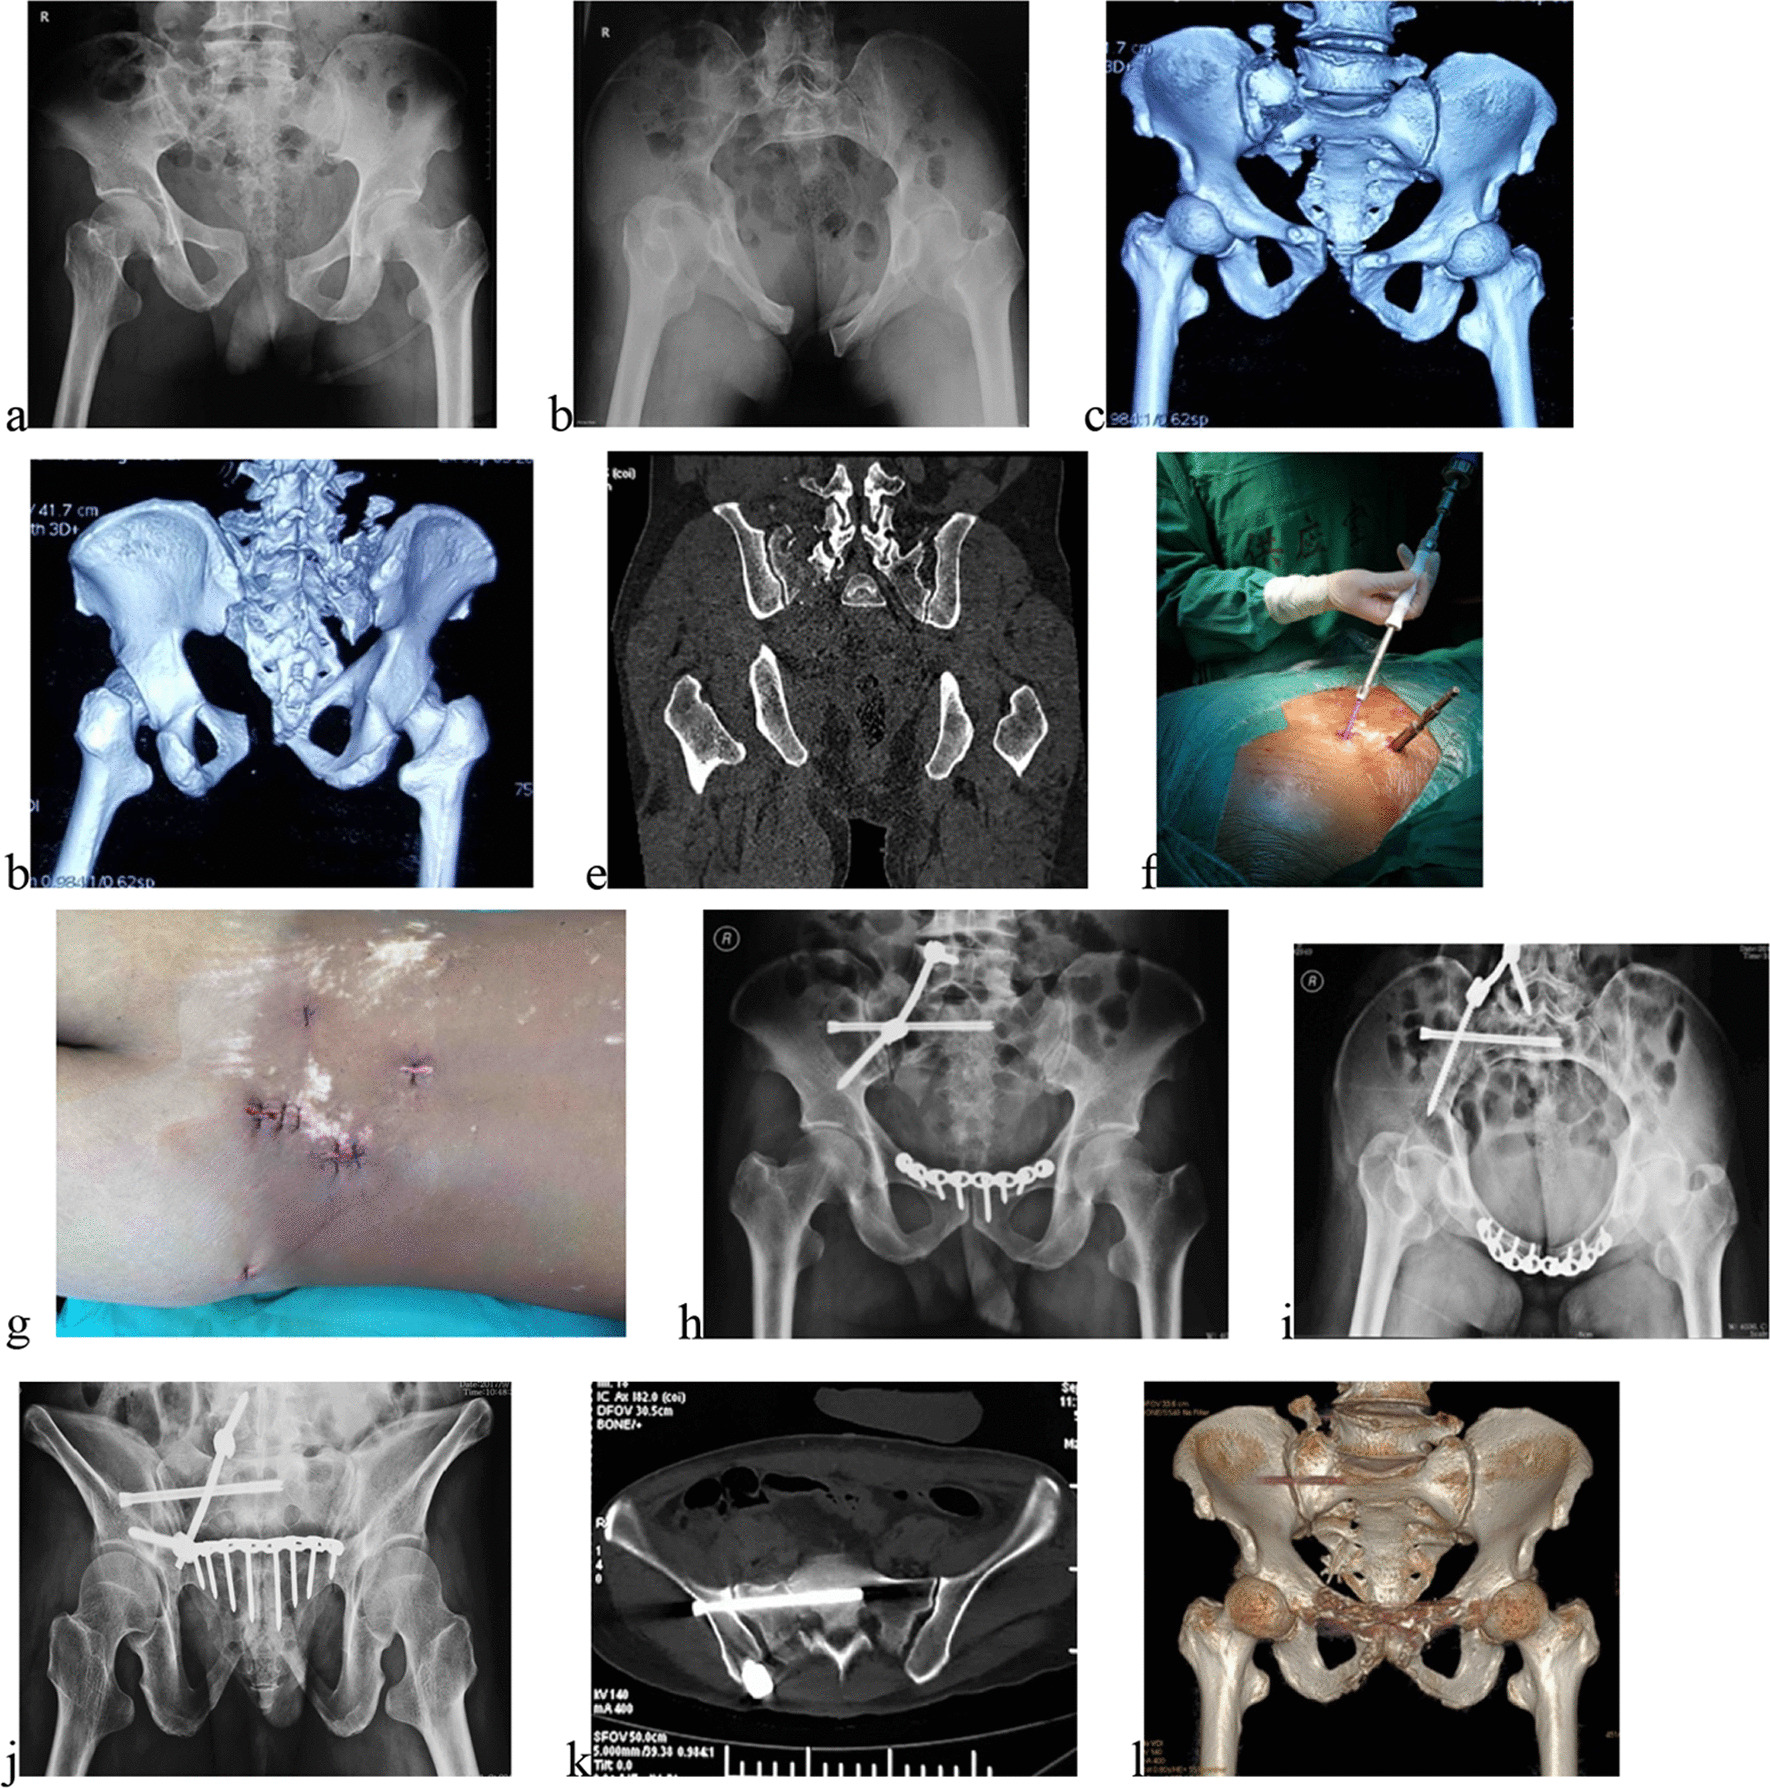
Male, 48 years old, a fall injury. a, b Anteroposterior and inlet pelvic views after admission. c, d 3D CT reconstruction: a Tile C1 pelvic ring injury. e The coronal view showing the right S1 facet fracture. f The percutaneous implantation of an iliac screw. g The sutured incisions. h–j Anteroposterior, inlet and outlet pelvic views after surgery. k The axial view revealing the good position of the iliosacral screw. l 3D CT reconstruction at one year demonstrating the union of the pelvic fracture
